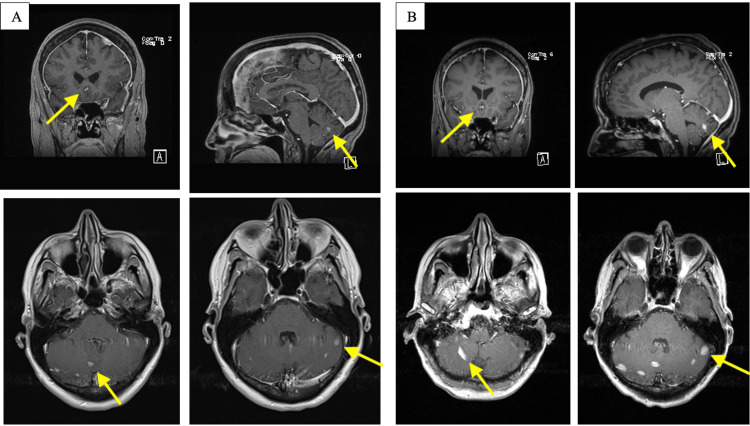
(A) Brain MRI obtained in January 2022 demonstrates pachymeningeal enhancement with a focal dural-based lesion in the left frontal lobe as well as multiple enhancing lesions in the posterior fossa. (B) Follow-up MRI obtained in March 2022 shows an increase in the size and number of brain lesions in the posterior fossa as well as worsening of the meningeal enhancement.
